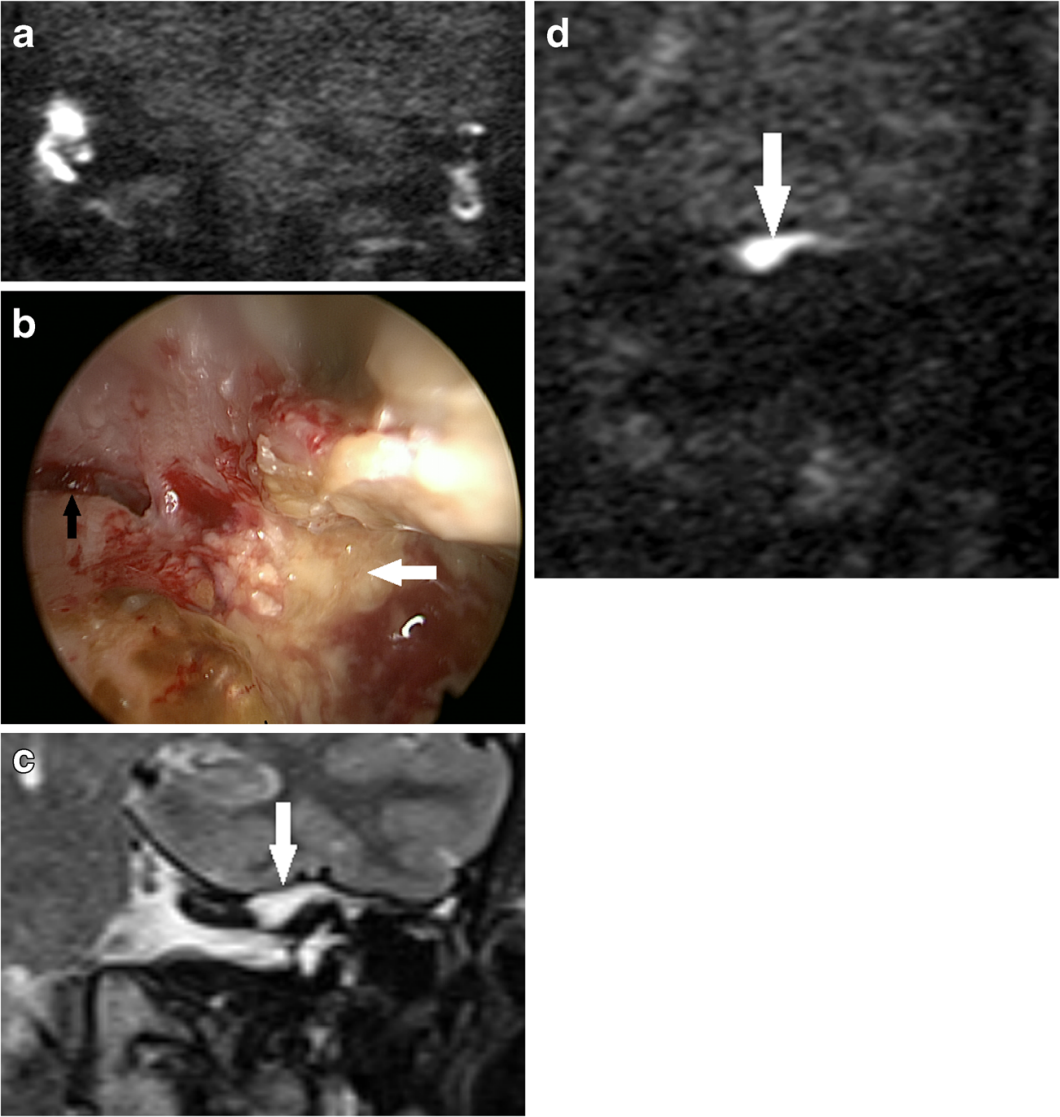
Using DWI following canal wall down surgery. In a patient who presented with persistent otorrhoea after CWD surgery, a the DWI b1000 images show high signal in the mastoid remnants bilaterally, which corresponded to infected debris/wax on clinical inspection. b On direct clinical inspection of the left mastoid remnant, which showed the infected debris/wax (*white arrow*), a bony defect (*black arrow*) is noted on the medial wall above the bony labyrinth. The defect communicated with extensive residual cholesteatoma hidden deep in the petrous apex, which was demonstrated by high signal soft tissue (*white arrow*) on the coronal c T2 weighted and d b1000 images
